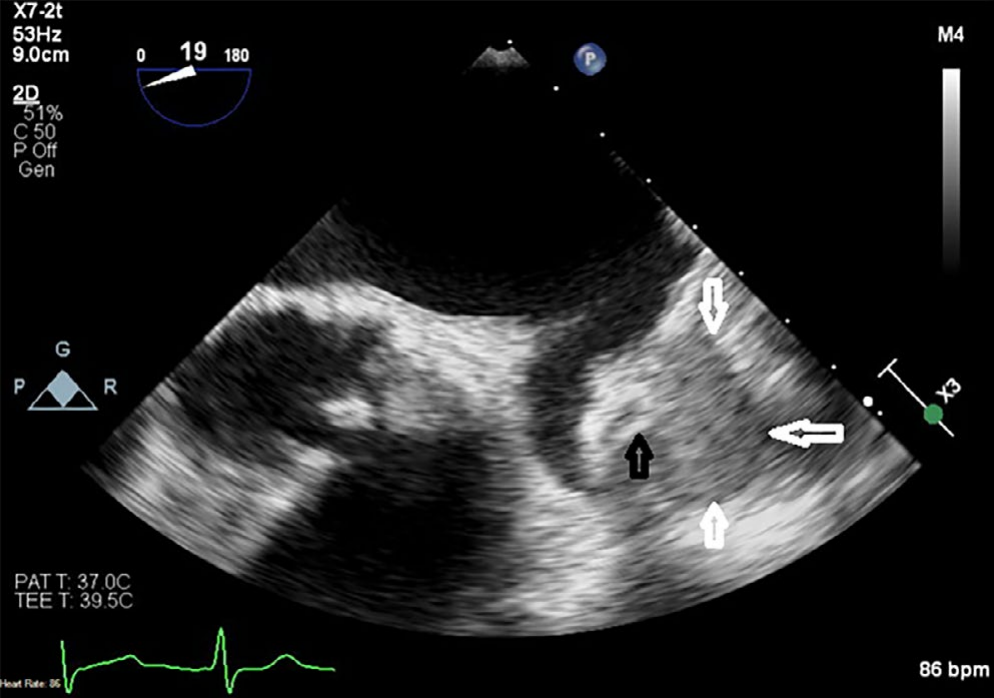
Transesophageal echocardiogram showing a 2.8 × 3.6 cm mass compressing left atrial appendage (white arrows) and saphenous vein graft stent (black arrow)
